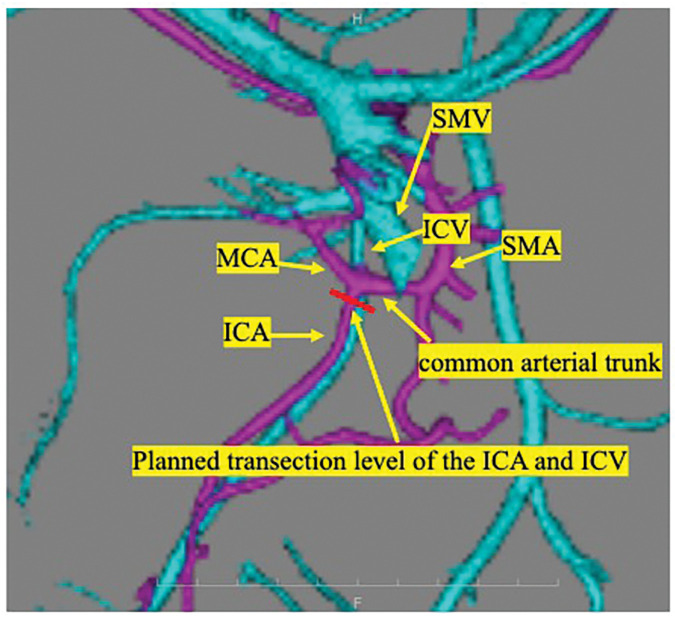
Preoperative CTA. Preoperative CTA demonstrating a common arterial trunk formed by the MCA and ICA. The ICV runs cranially to the common arterial trunk. The planned transection level of the ICA and ICV at the same level is indicated. ICA, ileocolic artery; ICV, ileocolic vein; MCA, middle colic artery; SMA, superior mesenteric artery; SMV, superior mesenteric vein
